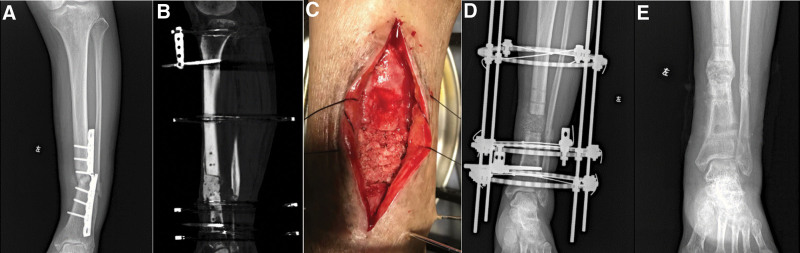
Case 8. A 64-yr-old male suffered infected nonunion and plate break of the left distal tibia. (A) Preoperative photograph. (B) The radiograph after the first stage. (C) Four weeks later the cement spacer was removed and the cancellous autografts were placed within the bone defect. (D) The radiograph after the second stage. (E) A radiograph showing the healing of the bone at 10 mo after the bone grafting.
